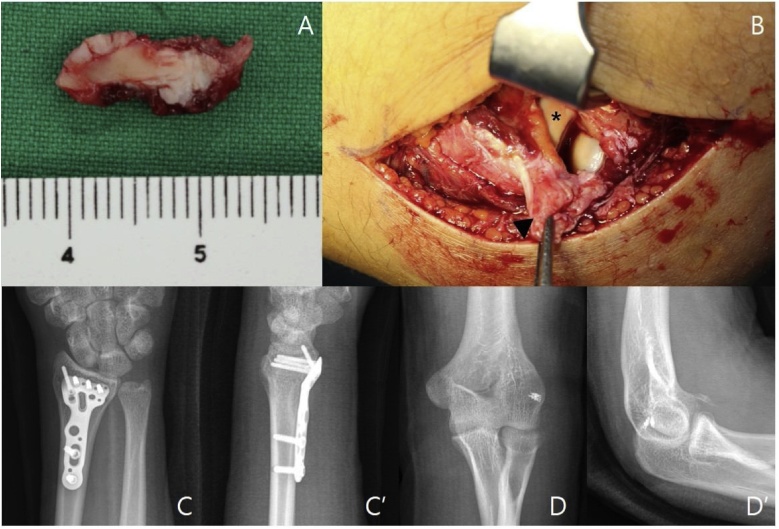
A, Intraoperative gross picture demonstrate intraarticular osseous body. B, Intraoperative gross picture demonstrate complete avulsion of the lateral collateral ligament (LCL) from the humeral attachment site was noticed along with partial avulsion of the common extensor origin and the annular ligament(Arrow head: lateral collateral ligament and common extensor origin, *: Radial head) C and C’, Immediate postoperative simple radiographs of the left wrist. D and D’, Immediate postoperative simple radiographs of the left elbow.
